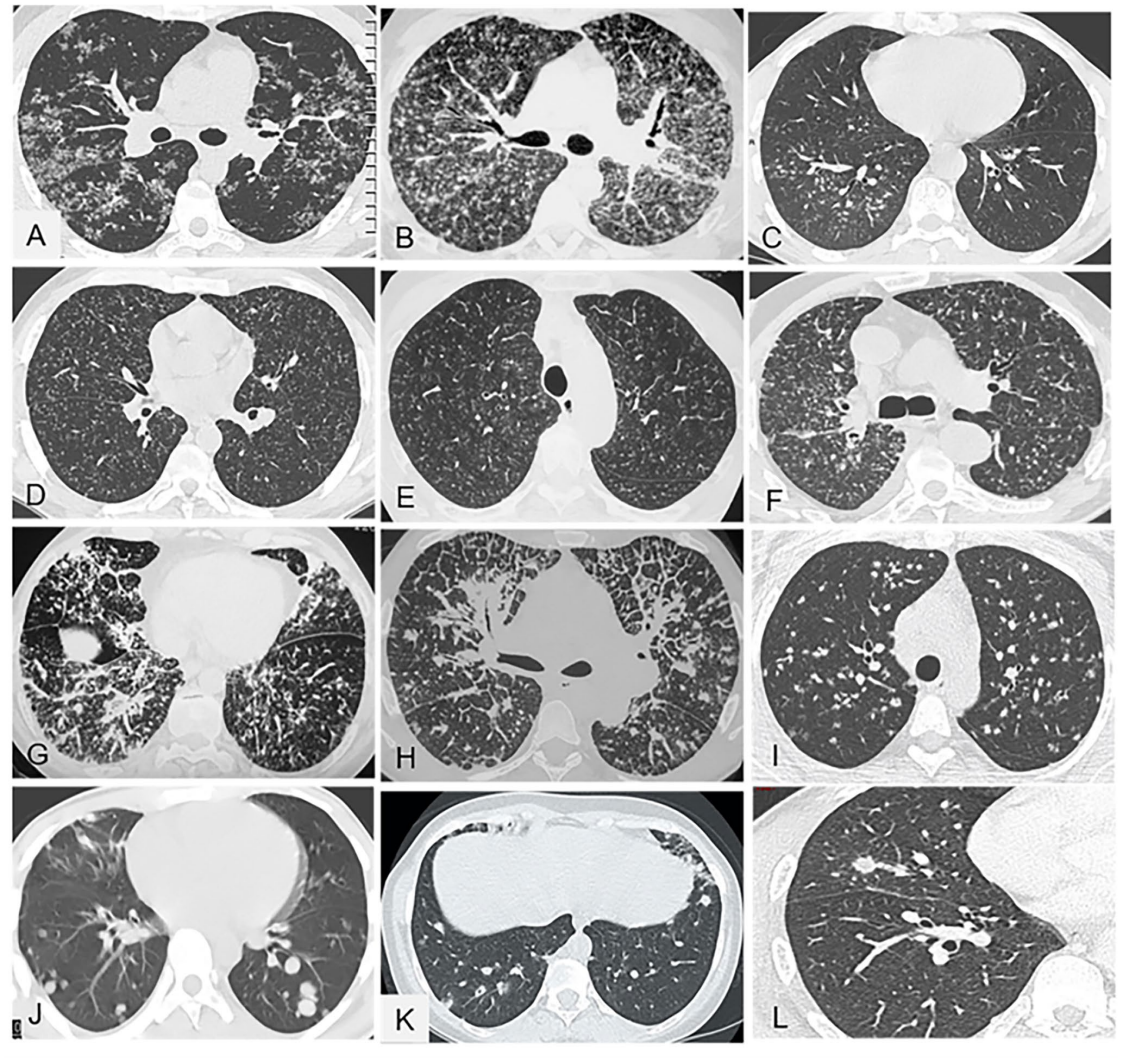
Representative of micronodular parenchymal pattern (A-H) and nodular pattern (I-L) in chest CT (parenchymal window). (A) Sarcoidosis with typical diffuse micronodules aggregated in small clusters and predominating along the fissures (lymphatic distribution). (B) Sarcoidosis: less typical presentation with profuse micronodules whose distribution is more difficult to determine; nevertheless, the predominance of micronodules along the fissures is suggestive of lymphatic distribution. (C) Bronchogenic tuberculosis with micronodules in a unilateral and centrilobular distribution that spares the pleural surface. (D) Typical, hematogenous, miliary tuberculosis with minute micronodules that are all the same size and are distributed uniformly (i.e., at random) throughout both lungs. (E) Hypersensitivity pneumonitis with profuse, minute, diffuse micronodules in a centrilobular distribution that spares the subpleural parenchyma, leaving a 2–3 cm uninvolved border. (F) Neoplastic miliary: the distribution is not symmetrical and the nodules are larger and more irregular than in miliary tuberculosis. (G) Systemic amyloidosis with diffuse pulmonary involvement and no lymphadenopathy; the micronodules are diffuse with no particular distribution and are combined with several irregular lines and thickened interlobular septa. (H) Lymphangitis carcinomatosa: thickened peribronchovascular interstitium, nodules, and nodular septal reticulations without distortion. (I) Pulmonary sarcoidosis: multiple nodules with irregular and well-defined contours. (J) Pulmonary metastases from renal carcinoma: sharply contoured nodules in a more peripheral distribution. (K) Granulomatosis-associated common variable immunodeficiency: nodules, some of which exhibit the halo sign. (L) Chronic histoplasmosis: sparse pulmonary nodules.
